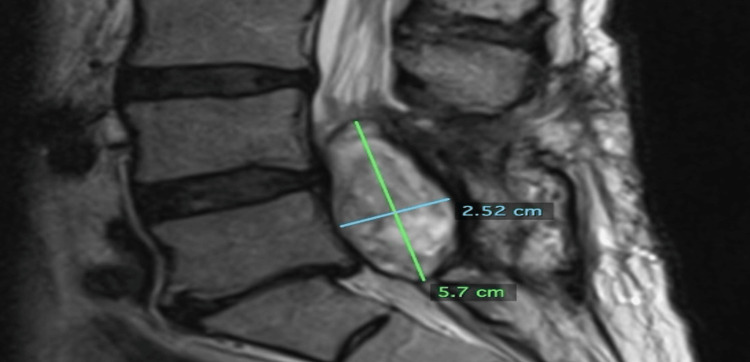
Sagittal image from an MRI L-spine with contrast dated November 16, 2022. The tumor measured 5.7 cm superiorly to inferiorly and 2.52 cm anteriorly to posteriorly.
